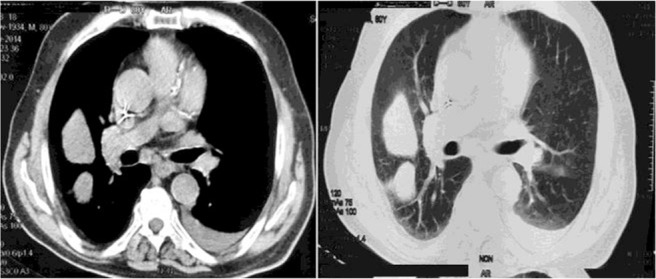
Computed tomography images showing bilateral plural effusion and three mass-like densities in the right lung
